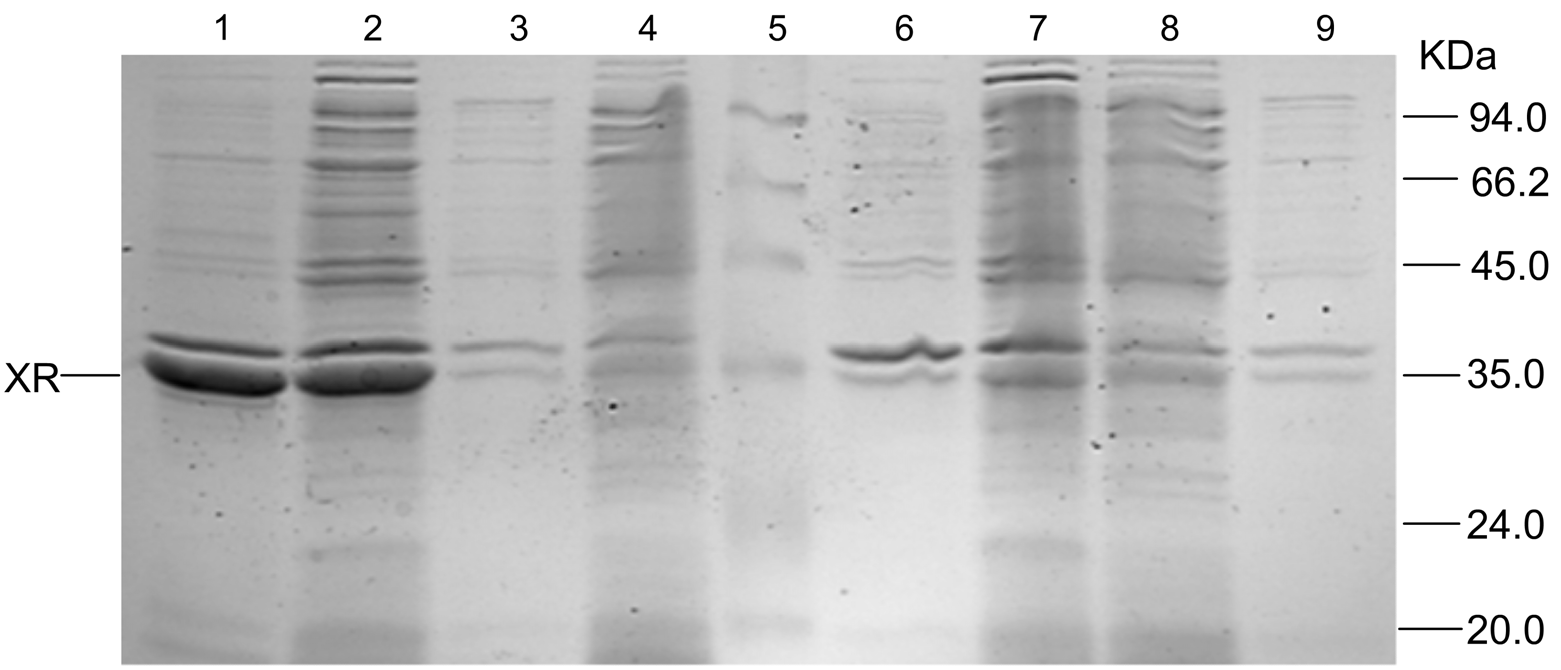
SDS-PAGE analysis of crude extracts of XR, NT-XR and CT-XR. Expression was carried out in *E. coli *cells in LB medium containing kanamycin (50 μg ml^-1^) and IPTG (1 mM). Crude extracts of different variants were adjusted to the same OD_600 _concentration (12.41 OD/ml) before they were prepared, and 15 μl was loaded in each lane. Lanes: 1, the insoluble fraction of NT-XR; 2, the soluble fraction of NT-XR; 3, the insoluble fraction of pET30a; 4, the soluble fraction of pET30a; 5, marker; 6, the insoluble fraction of XR; 7, the soluble fraction of XR; 8, the soluble fraction of CT-XR; 9, the insoluble fraction of CT-XR.
